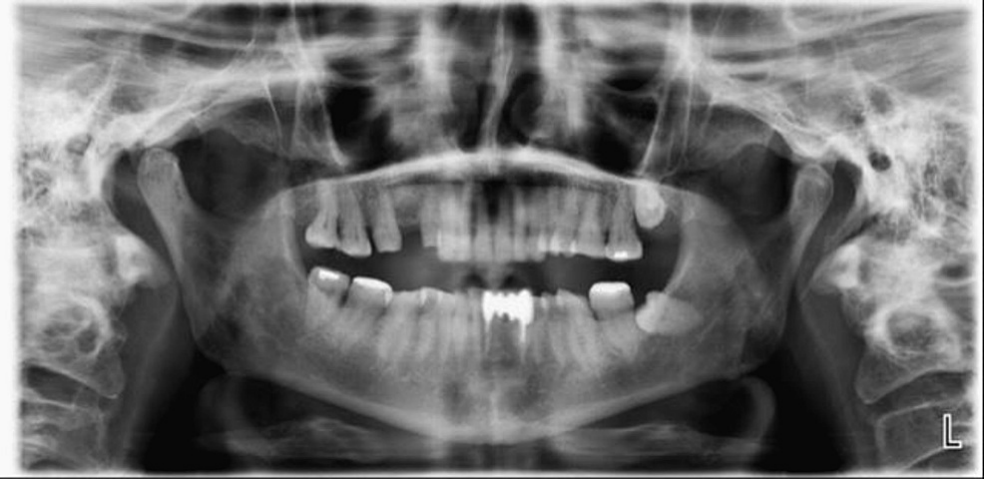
OPG image after completion of post and core Orthopantomography (OPG) image showing the preoperative status of tooth wear.
